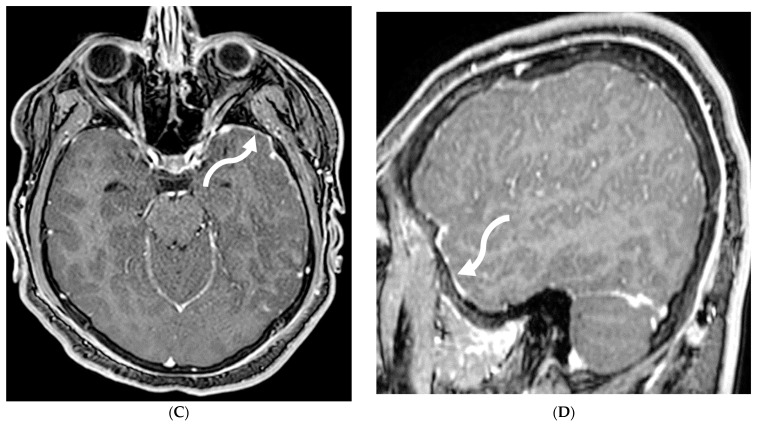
Axial T2 (A), post contrast FLAIR (B), axial and sagittal (left) T1 (C,D). 16-year-old male with 3 weeks of headache, photophobia and vomiting. There is asymmetric left cerebral swelling with cortical T2 hyperintensity (white arrows) and anterolateral left temporal LME (curved arrows). Diagnosis: NMDA receptor encephalitis (initially thought to be HSV).
